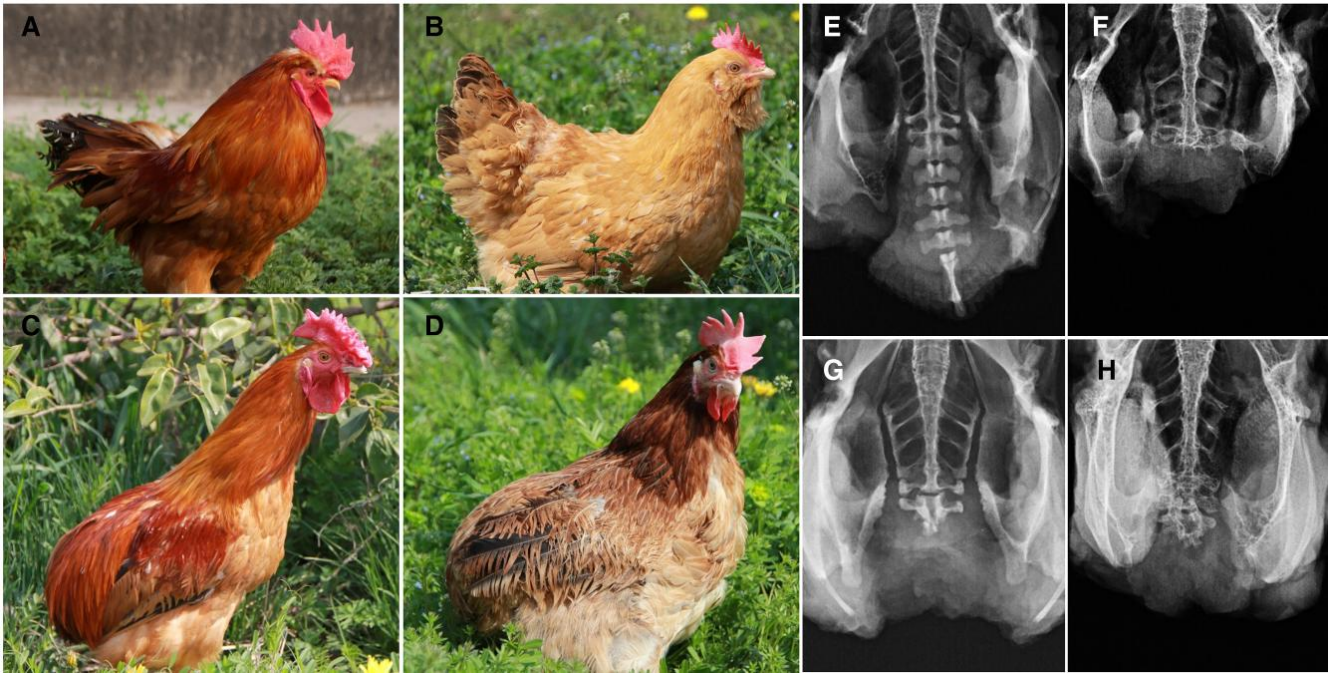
The rumpless Piao chicken. The WT cock (A), hen (B), Piao rumpless cock (C), and hen (D) are pictured, together with X-rays showing the tailbones of a WT (E) and 3 degrees of the rumpless trait (F, G, H) displayed in the Piao breed.
